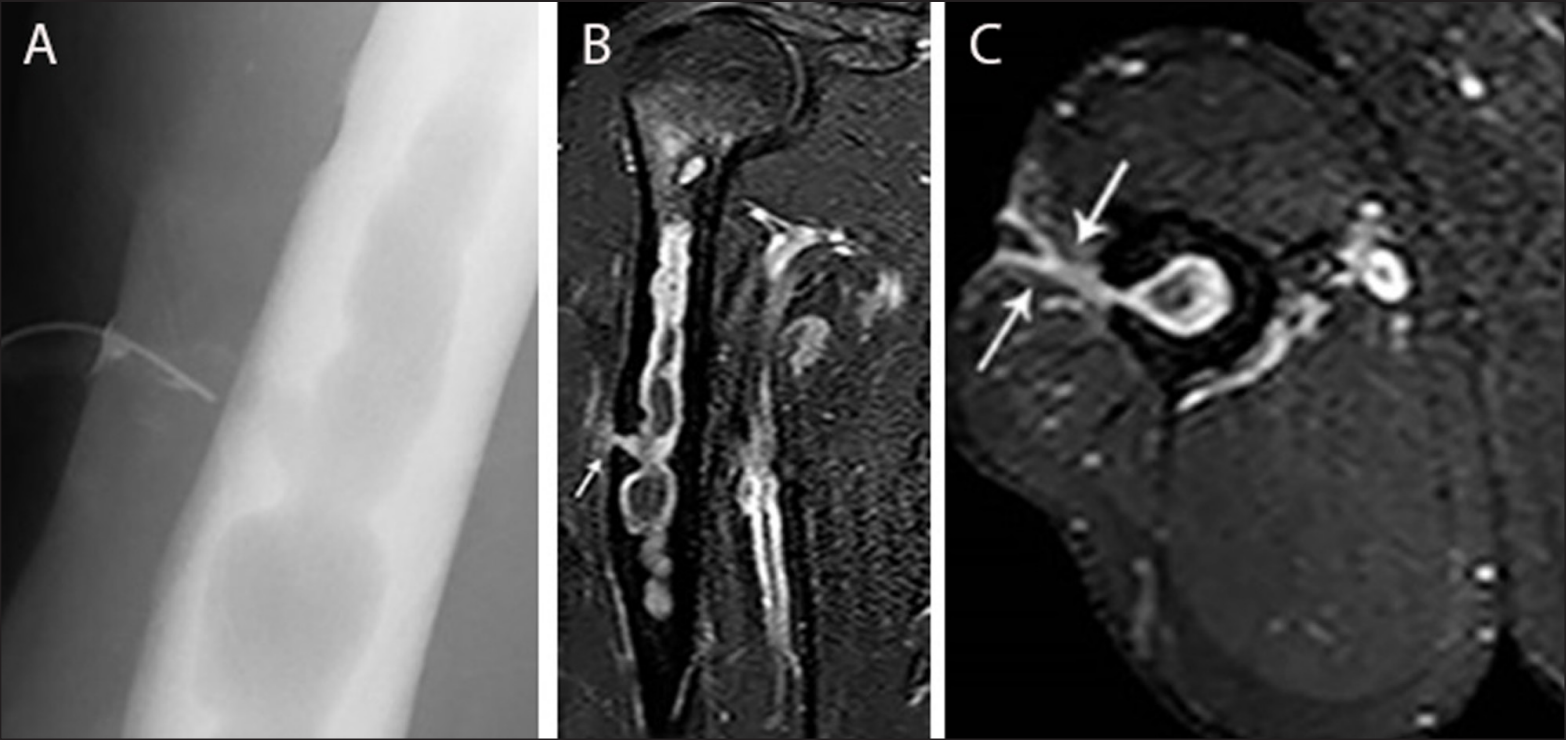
Chronic osteomyelitis of the right humerus with fistula formation. Plain radiograph (sinography) (a), coronal (b) and axial (c) Fat–Sat T1–WI after gadolinium contrast administration. The sinography (a) shows an intramedullary well defined lytic lesion with scalloping of the cortex. Note the presence of a catheter in the sinus. There is enhancement of the wall of the intra-osseous abscess and the wall of the fistula (white arrow) (b, c). Image courtesy Dr. H. Declercq, Dendermonde.
